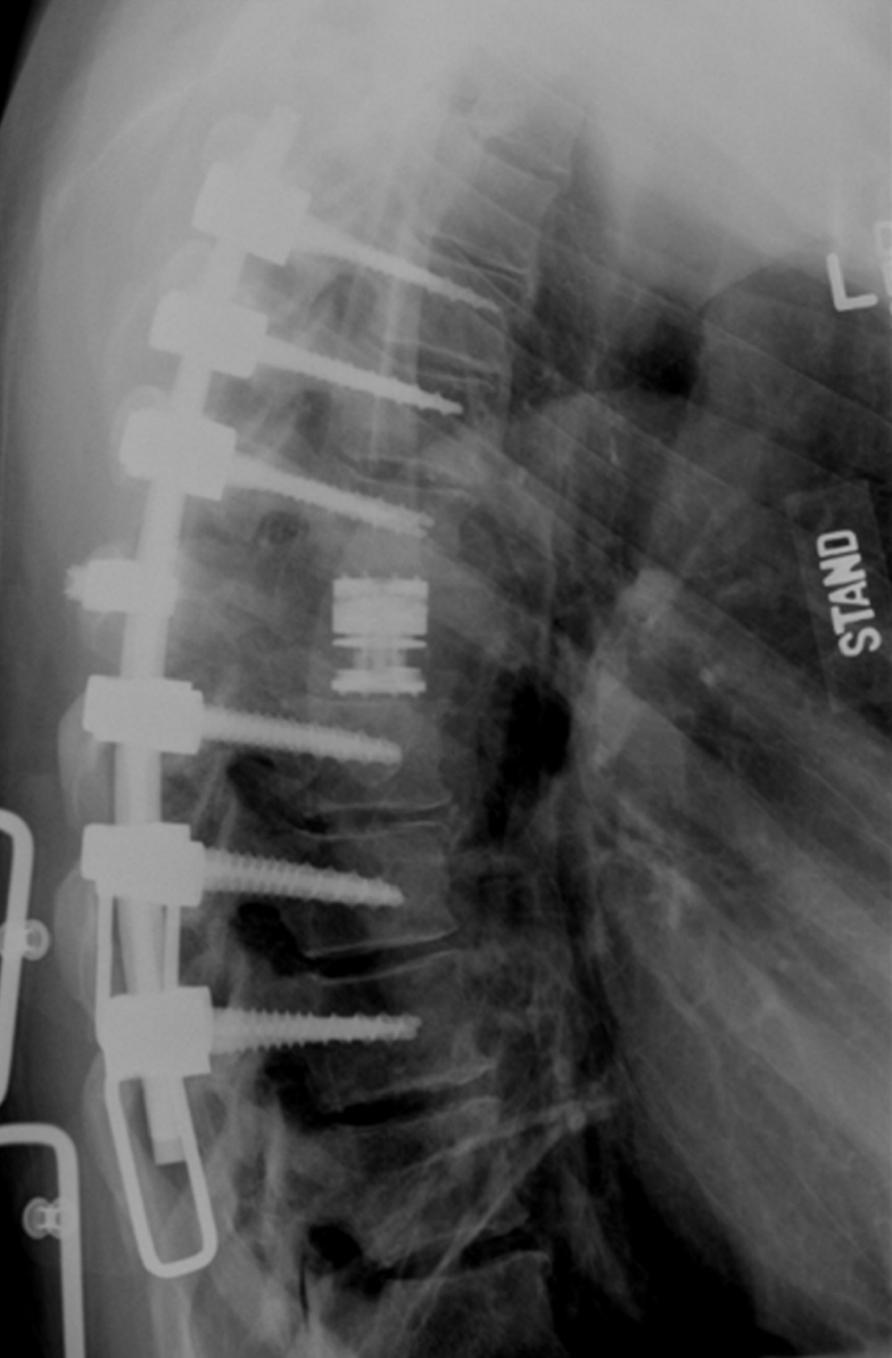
Postoperative upright lateral thoracic x-ray Screws T4 through T10, skipping T7. Expandable cage and a single cross-link at T7. Maintenance of the patient’s anatomical kyphotic curve is demonstrated.
